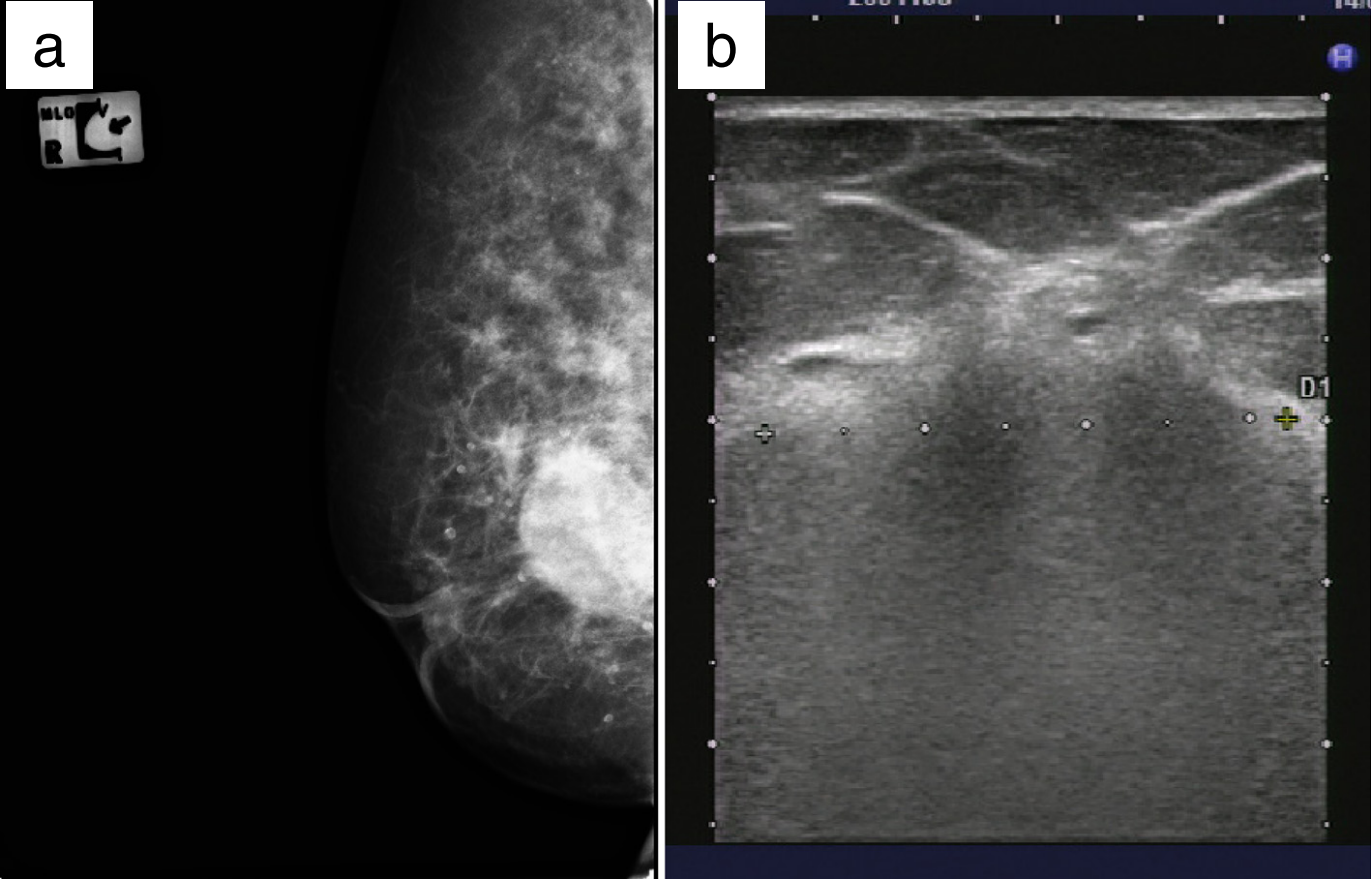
Mammography and ultrasonography. (a) Mammography showed a smooth mass that retracted the right nipple. (b) Ultrasonography revealed a so-called ‘snowstorm’ appearance with diffuse hyperechogenic lesions and posterior shadowing.
